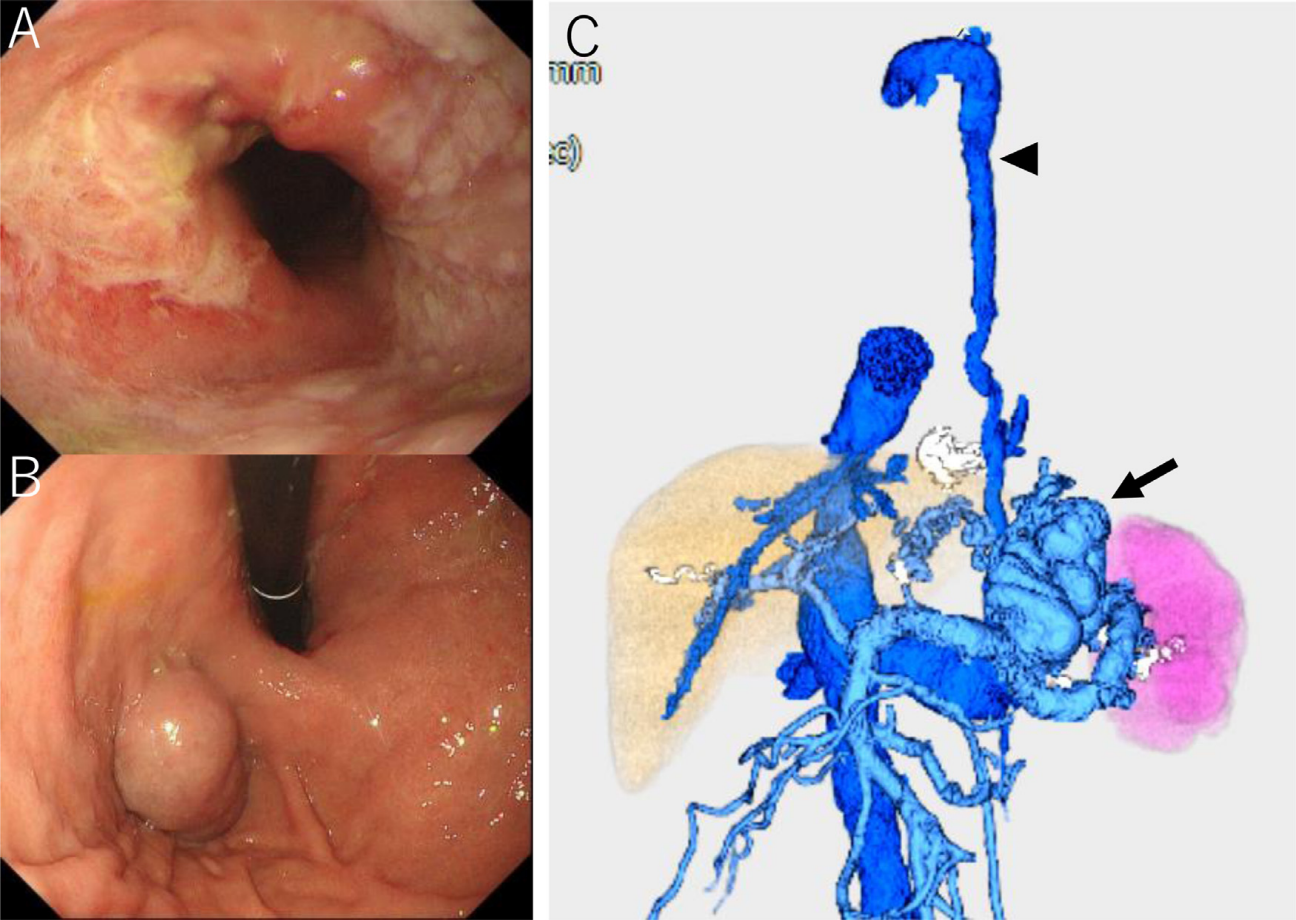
Endoscopic picture and 3D-CT reconstruction image after treatment. (A) Endoscopic picture 3 weeks after PTO shows disappearance of esophageal varices. (B) Endoscopic picture 3 weeks after PTO shows no change of gastric varices. (C) 3D-CT reconstruction image 30 months after PTO shows the disappearance of esophageal varices. The gastrorenal shunt and azygos vein are not affected. The S/L ratio is 0.3.
